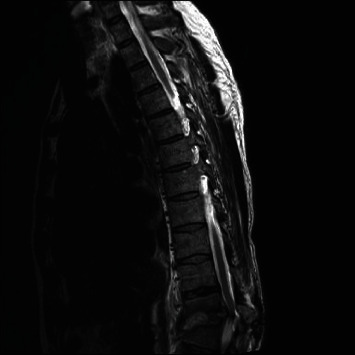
MR thoracic spine T2-weighted image 1 month later showing progressive collapse of T11 and T12 vertebral bodies and severe spinal stenosis.
